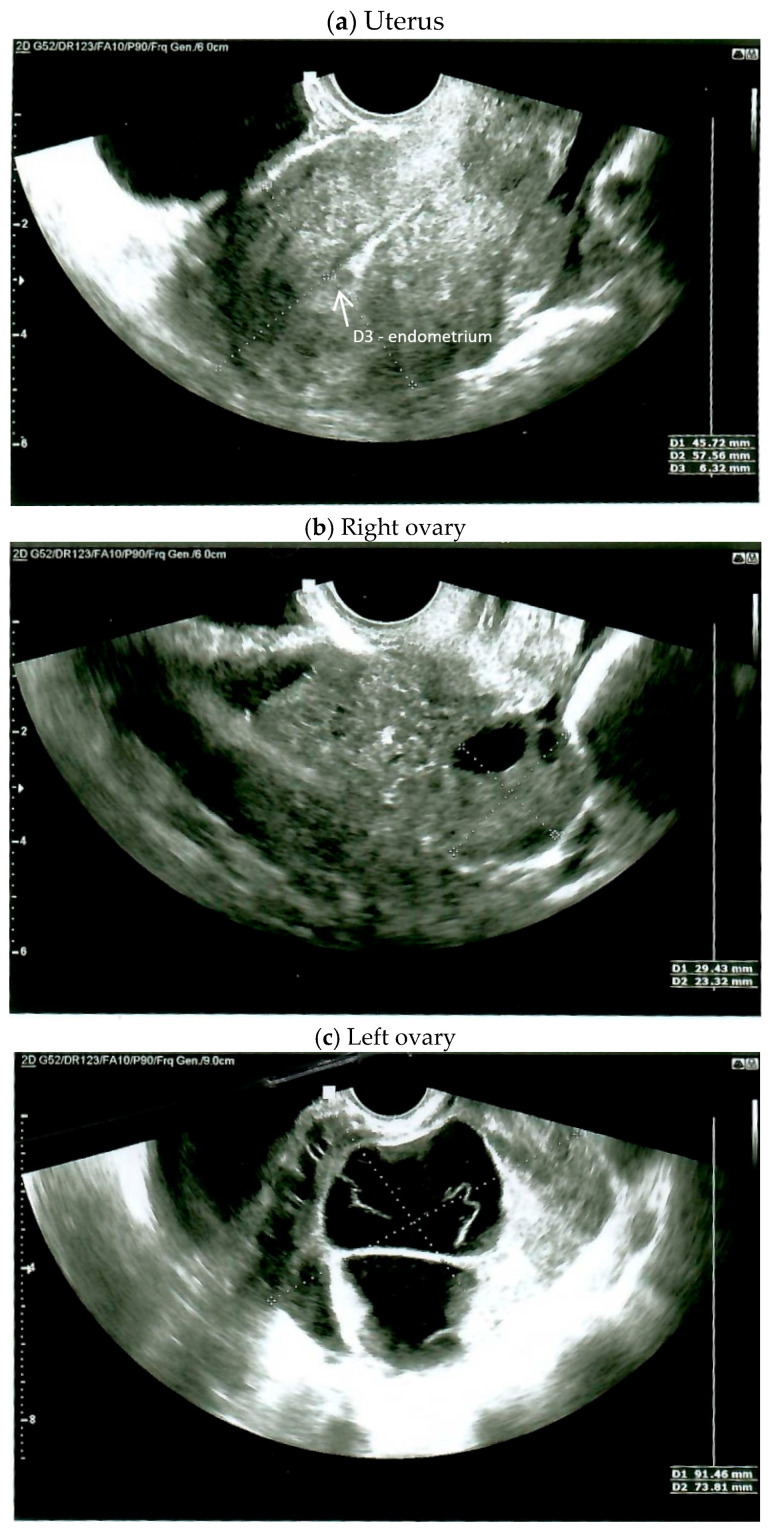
Ultrasound on the day of admission.
